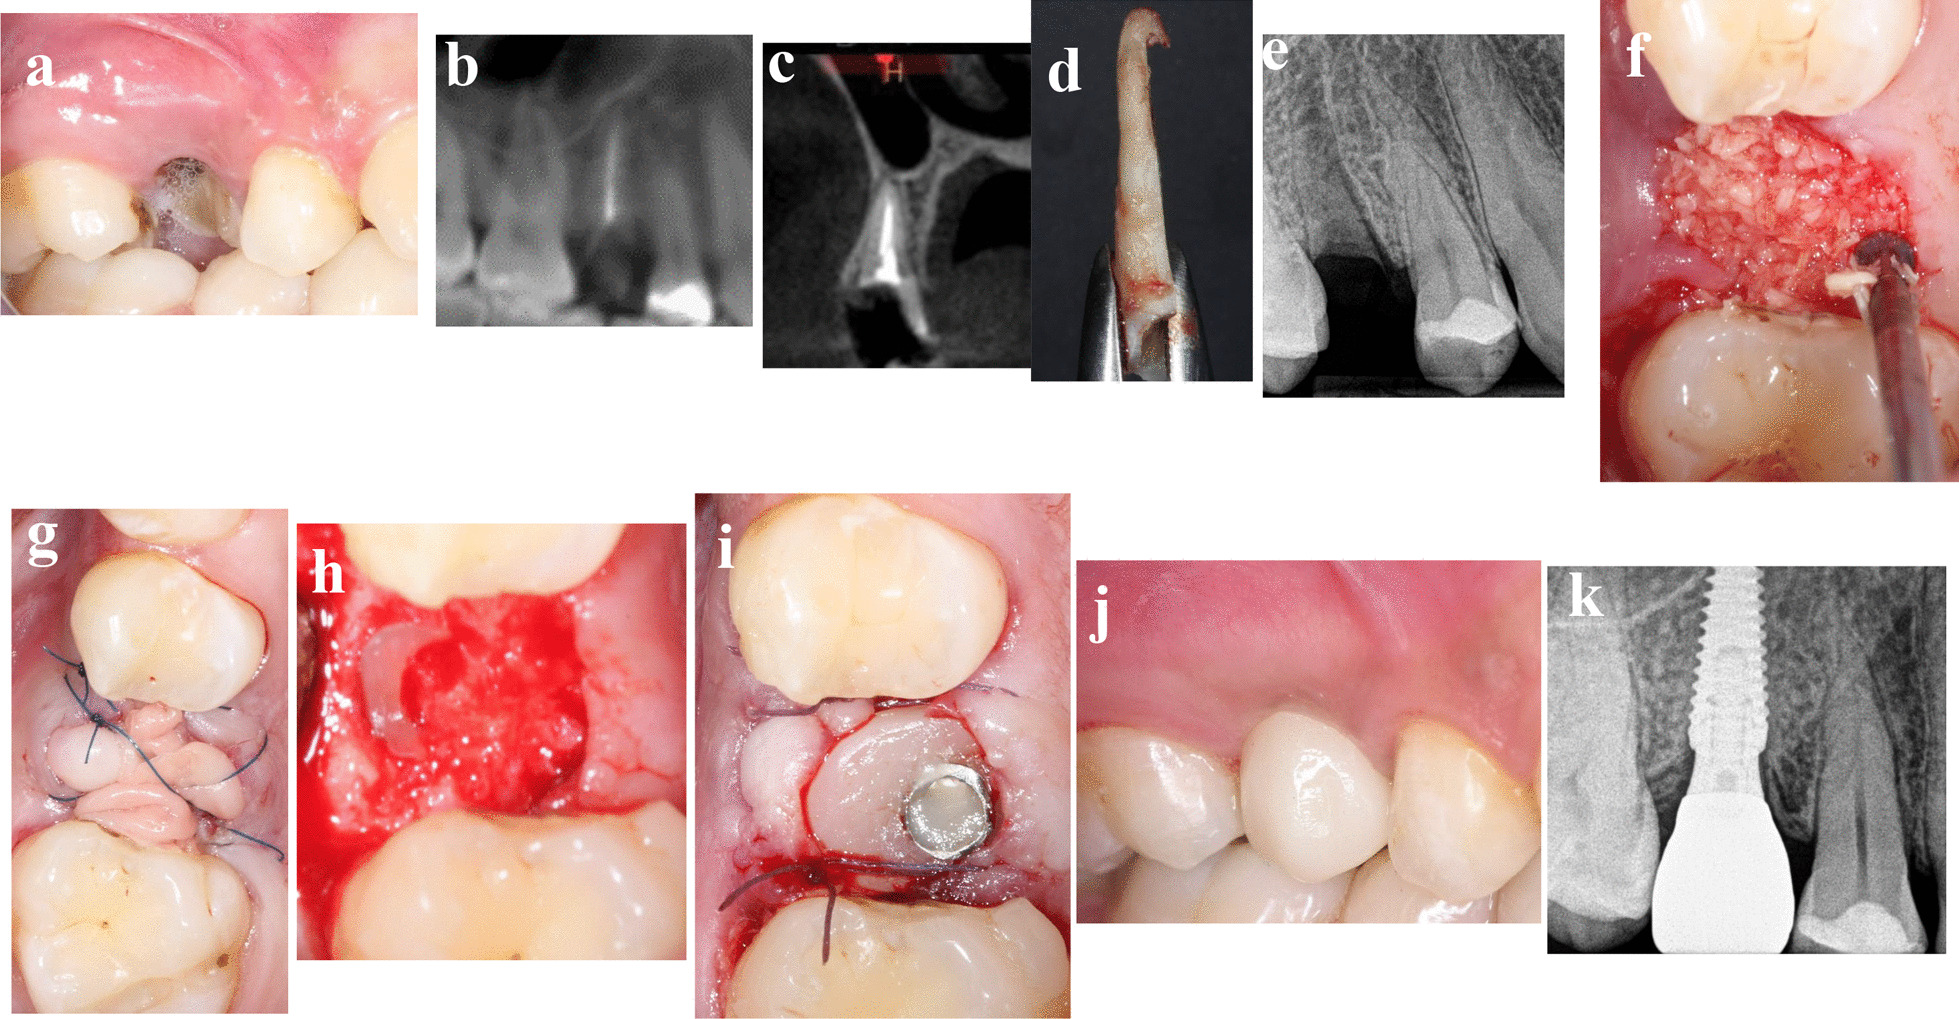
a–k Photos and radiographs of case #2 replacing maxillary right second premolar. a Preoperative buccal view; b panoramic view of preoperative CBCT; c cross-sectional view of preoperative CBCT; d the extracted palatal portion with the apex; e periapical radiograph after shield preparation; f FDBA particles filled the socket; g PRF membrane sealed the socket; h the healed socket at reentry of 6 months; i customized healing abutment fitted immediately after implant placement; j buccal view of the final implant restoration at 13 months post-loading; k periapical radiograph at 13 months post-loading
